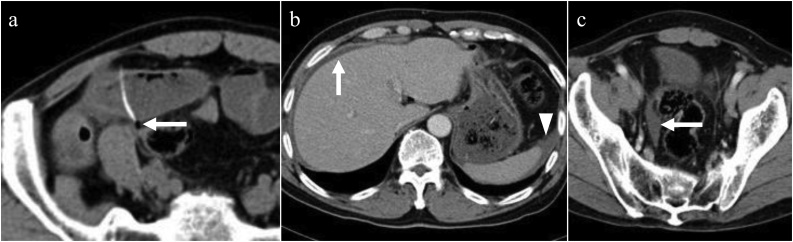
Findings from the computed tomography scan of the abdomen in Case 1. (a) A linear hyperdense foreign body (FB) inside the ileal lumen and free abdominal air adjacent to the FB (arrow). (b) Ascites around the liver (arrow) and the spleen (arrowhead). (c) Ascites in the pelvic cavity (arrow).
